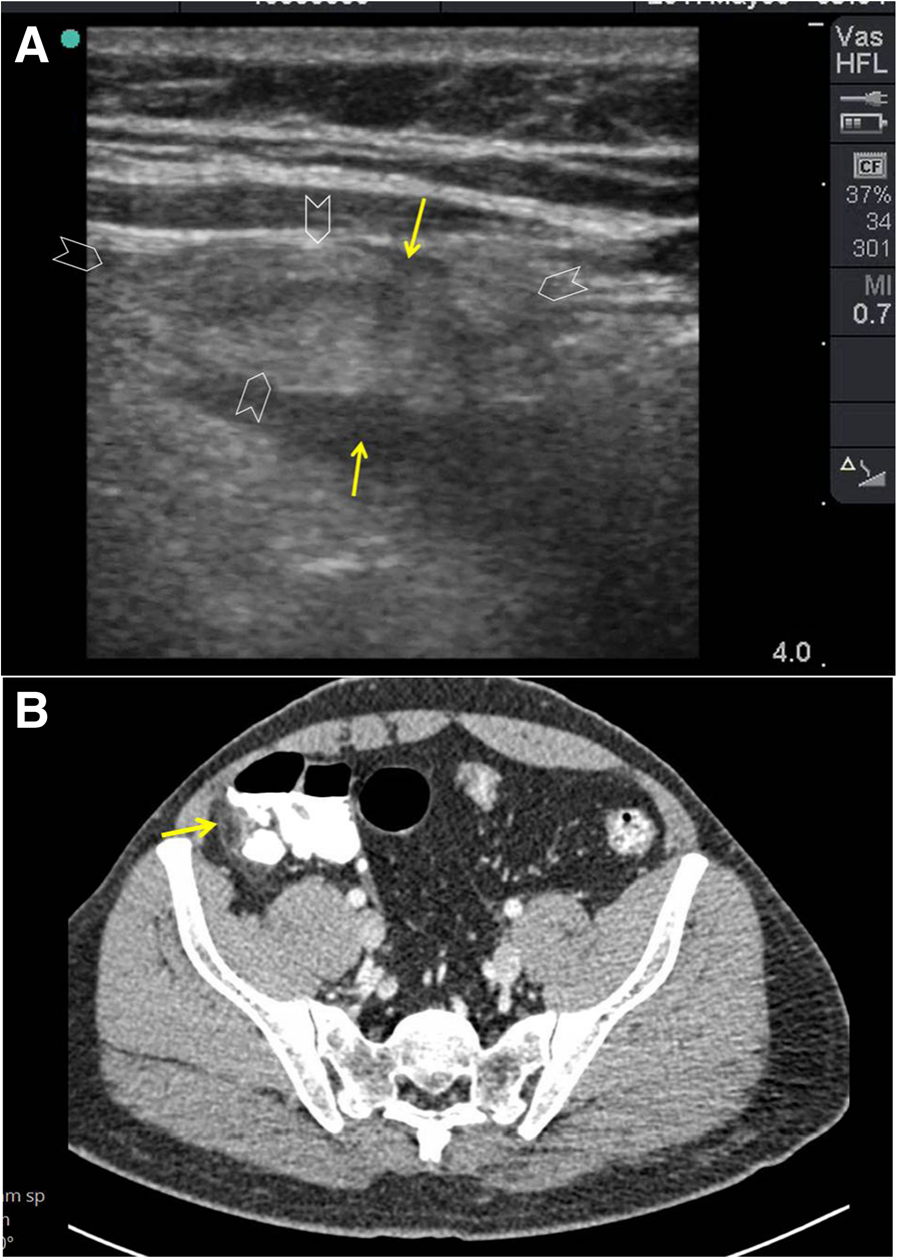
A 36-year-old man presented with right iliac fossa pain. The patient was afebrile. There was no leukocytosis and C-reactive protein was normal. The abdomen was soft but tender. POCUS (a) using a high-frequency linear probe showed a hyperechoic non-compressible ovoid mass adherent to the colonic wall at the maximum tenderness point (arrowheads) which was surrounded by fluid (yellow arrows). Abdominal CT scan with intravenous contrast (b) confirmed the diagnosis of epiploic appendagitis (yellow arrow)
